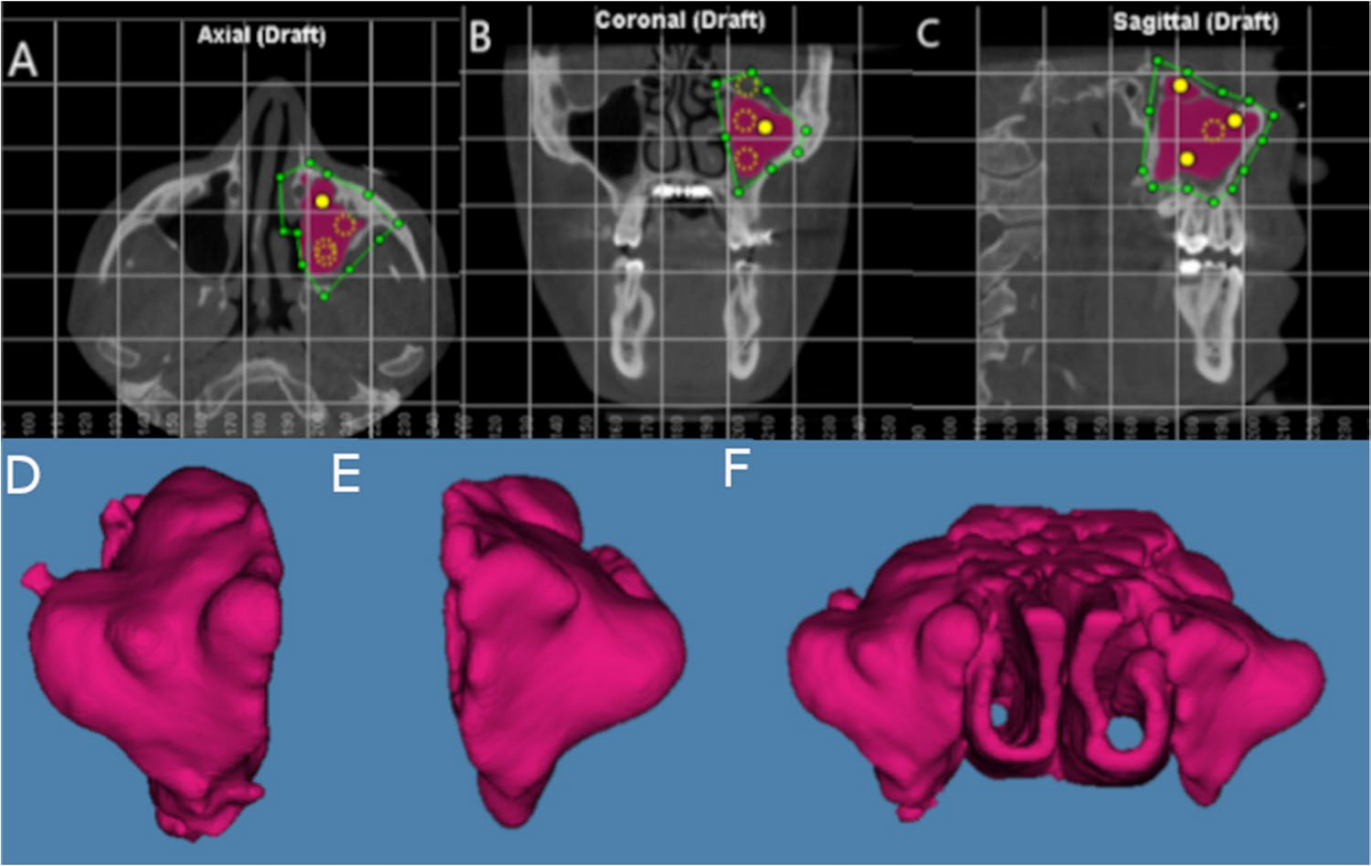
A Axial, B coronal, and C sagittal plane of the CBCT scans. The green line indicates the selected area, yellow points represent the air density, and the purple area shows the volume airway of the left maxillary sinus. D Volumetric assessment of the right maxillary sinus, E left maxillary sinus, and F nasal and maxillary sinus airway complex
